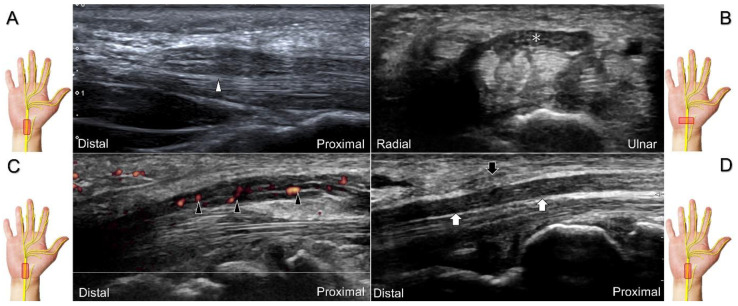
Sonographic images of patients with carpal tunnel syndrome, showing focal swelling proximal to the compression site (A), flattening at the compression site (B), intraneural hypervascularity (C), and loss of the trimline pattern (D). White arrowhead: focal swelling of the median nerve; asterisk: flattening of the median nerve; black arrowheads: intraneural hypervascularity of the median nerve; white arrows: loss of the trimline pattern of the median nerve; black arrow: thickened flexor retinaculum.
